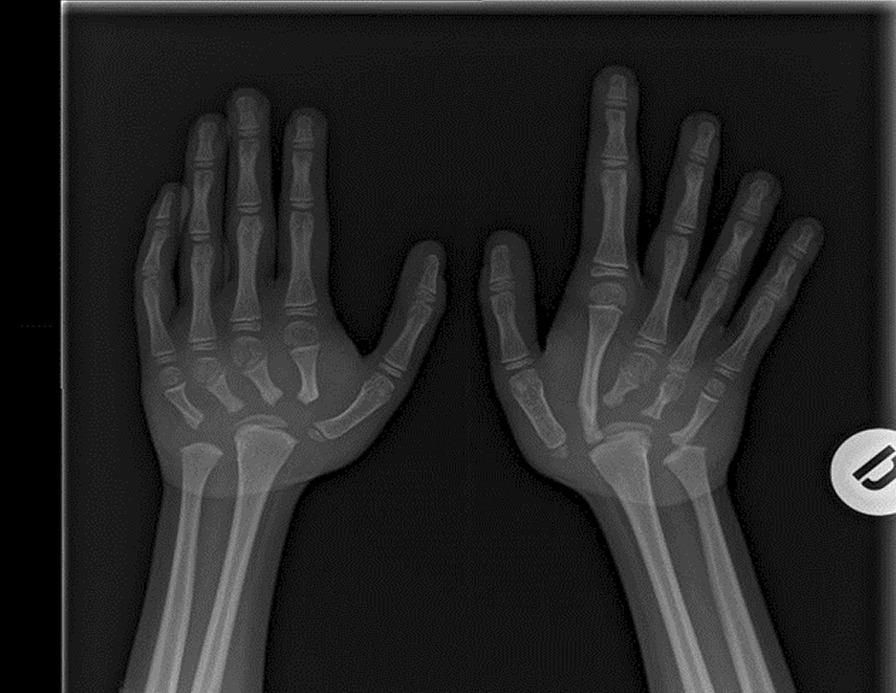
Pt. 6 X-ray at age 9y 11m. Bilateral hand radiograph showing complete resorption of carpal bones and generalized osteopenia
